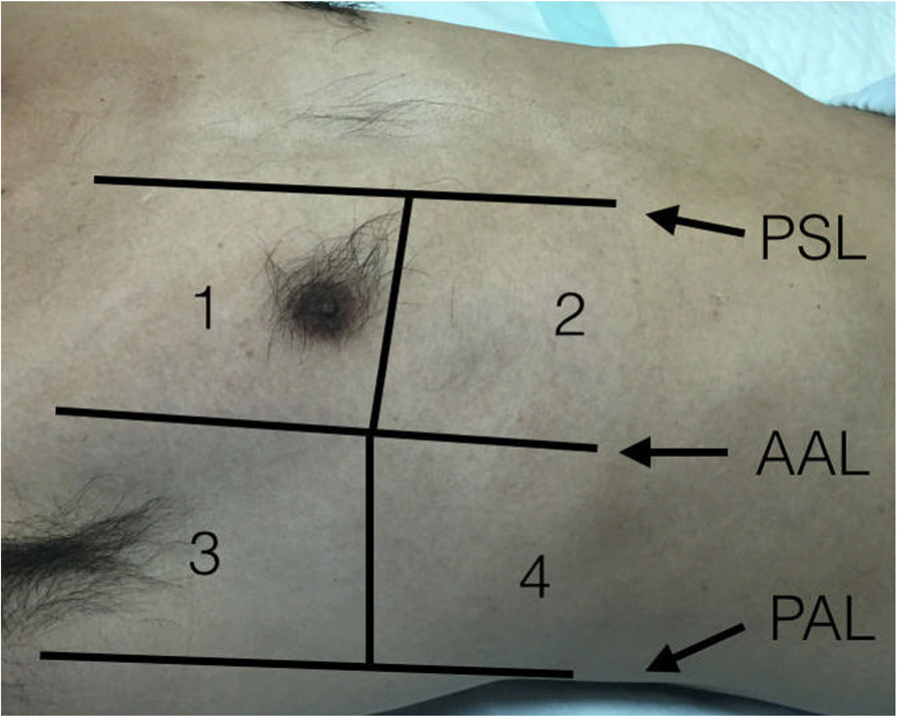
The four chest areas per side considered for complete twelve- zone lung ultrasound examination. Areas 1 and 2 denote the upper anterior and lower anterior chest areas, respectively. Areas 3 and 4 denote the upper lateral and basal lateral chest areas, respectively. PSL parasternal line, AAL anterior axillary line, PAL posterior axillary line.
